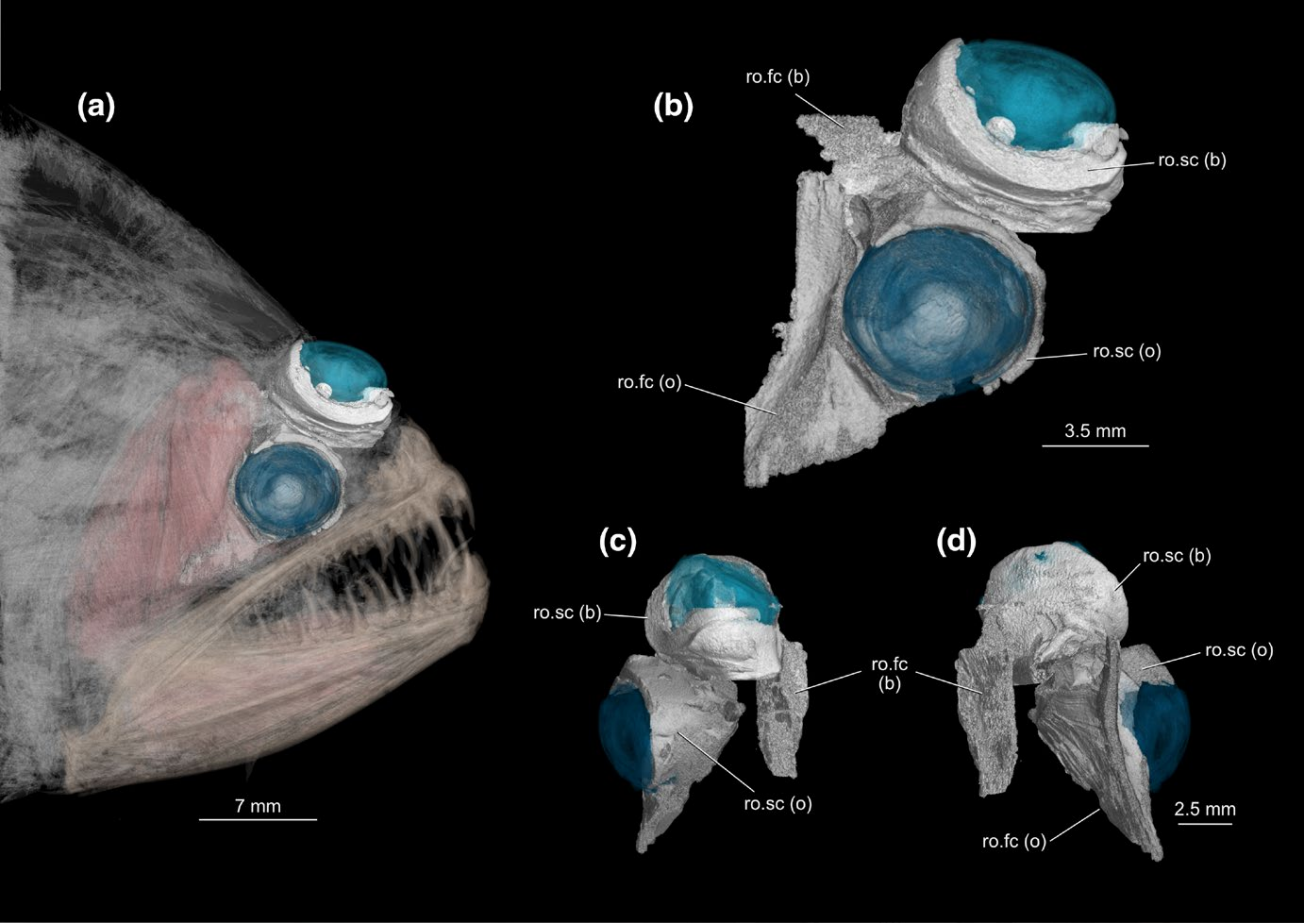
X‐ray microcomputed tomographies of the head of *Psettodes erumei* showing the position of the *recessus orbitalis* of both the ocular and blind sides. (a) Ocular side of the head, (b) ocular side of the eyes and their *recessus orbitales*, (c) frontal view, (d) posterior view. (b) blind side; (o) ocular side; ro.fc, *recessus orbitalis*, facial chamber; ro.sc, *recessus orbitalis*, scleral chamber.
